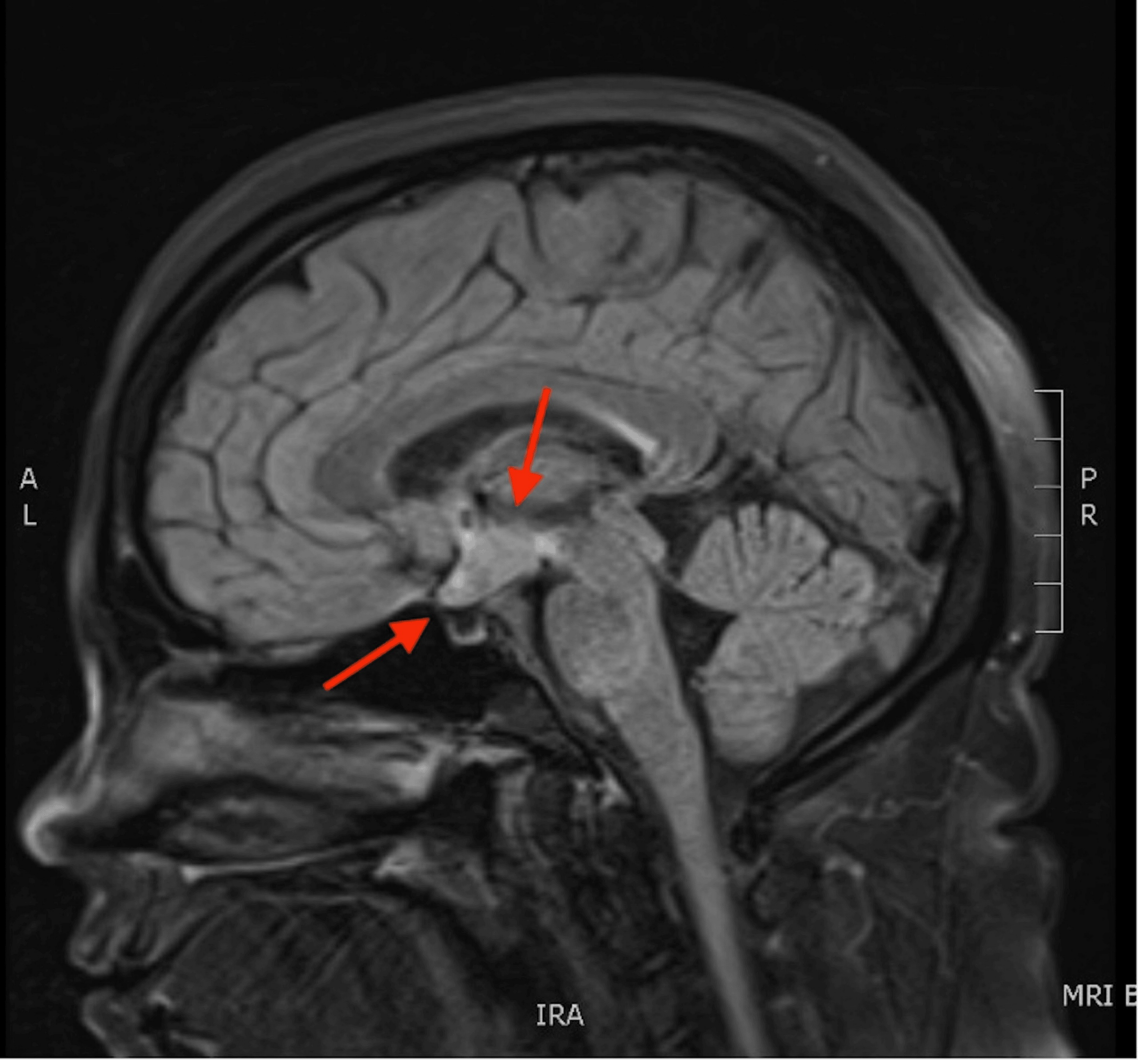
Magnetic resonance imaging in the sagittal plane demonstrating an enhancing hypothalamic mass measuring 2.4 cm x 1.7 cm x 1 cm with thickening of the optic chiasm.
